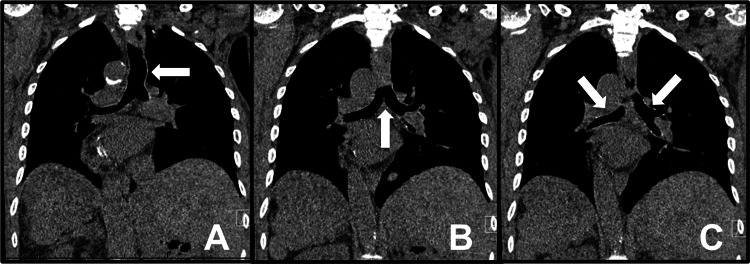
Appreciation of bronchial tree angulation. Ventral to dorsal CT images demonstrating by arrows the Trachea (A), Carina (B), and Right and Left primary bronchi (C). Left Primary Bronchi can be appreciated to have a more downward slope compared to the horizontal presentation seen on the Right Primary Bronchi in SIT.
